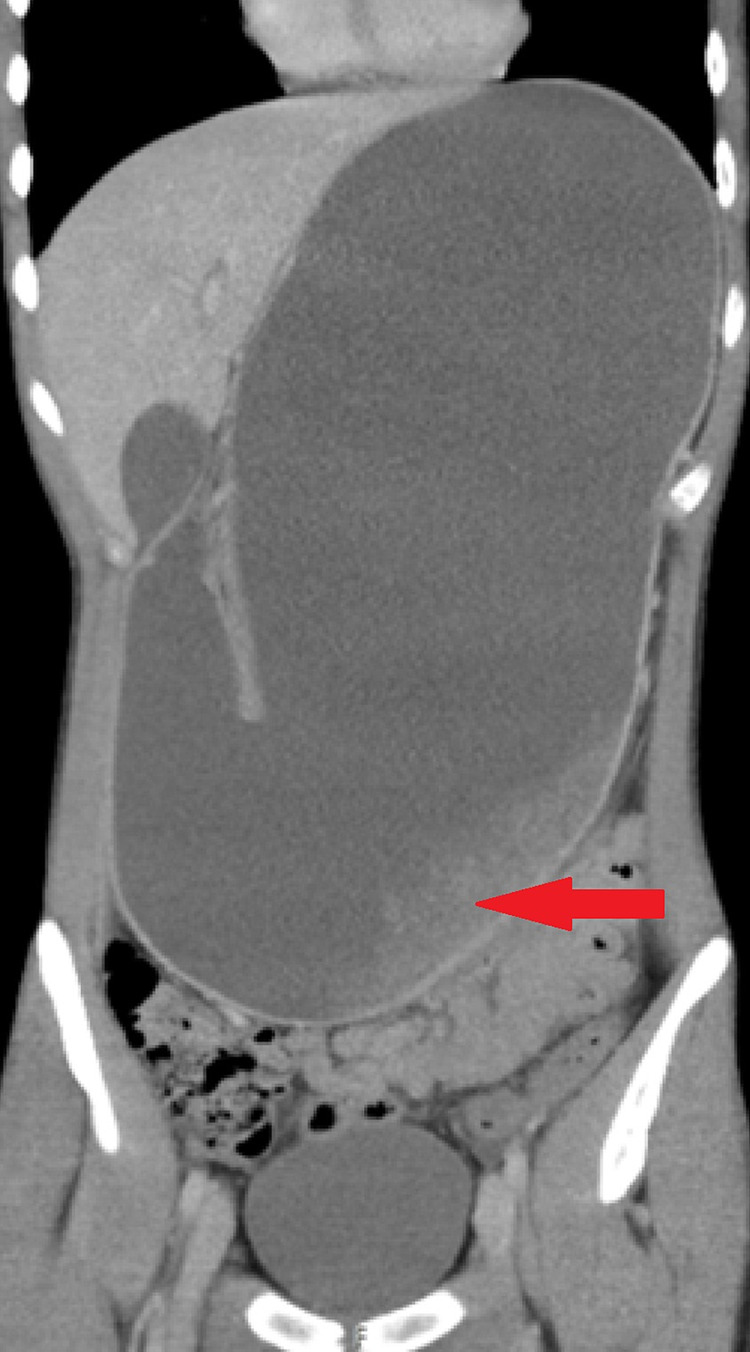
CT scan showing grossly distended stomach. The duodenum is not visible. Hazy intra-gastric opacification (red arrow) is likely to be food residue.
